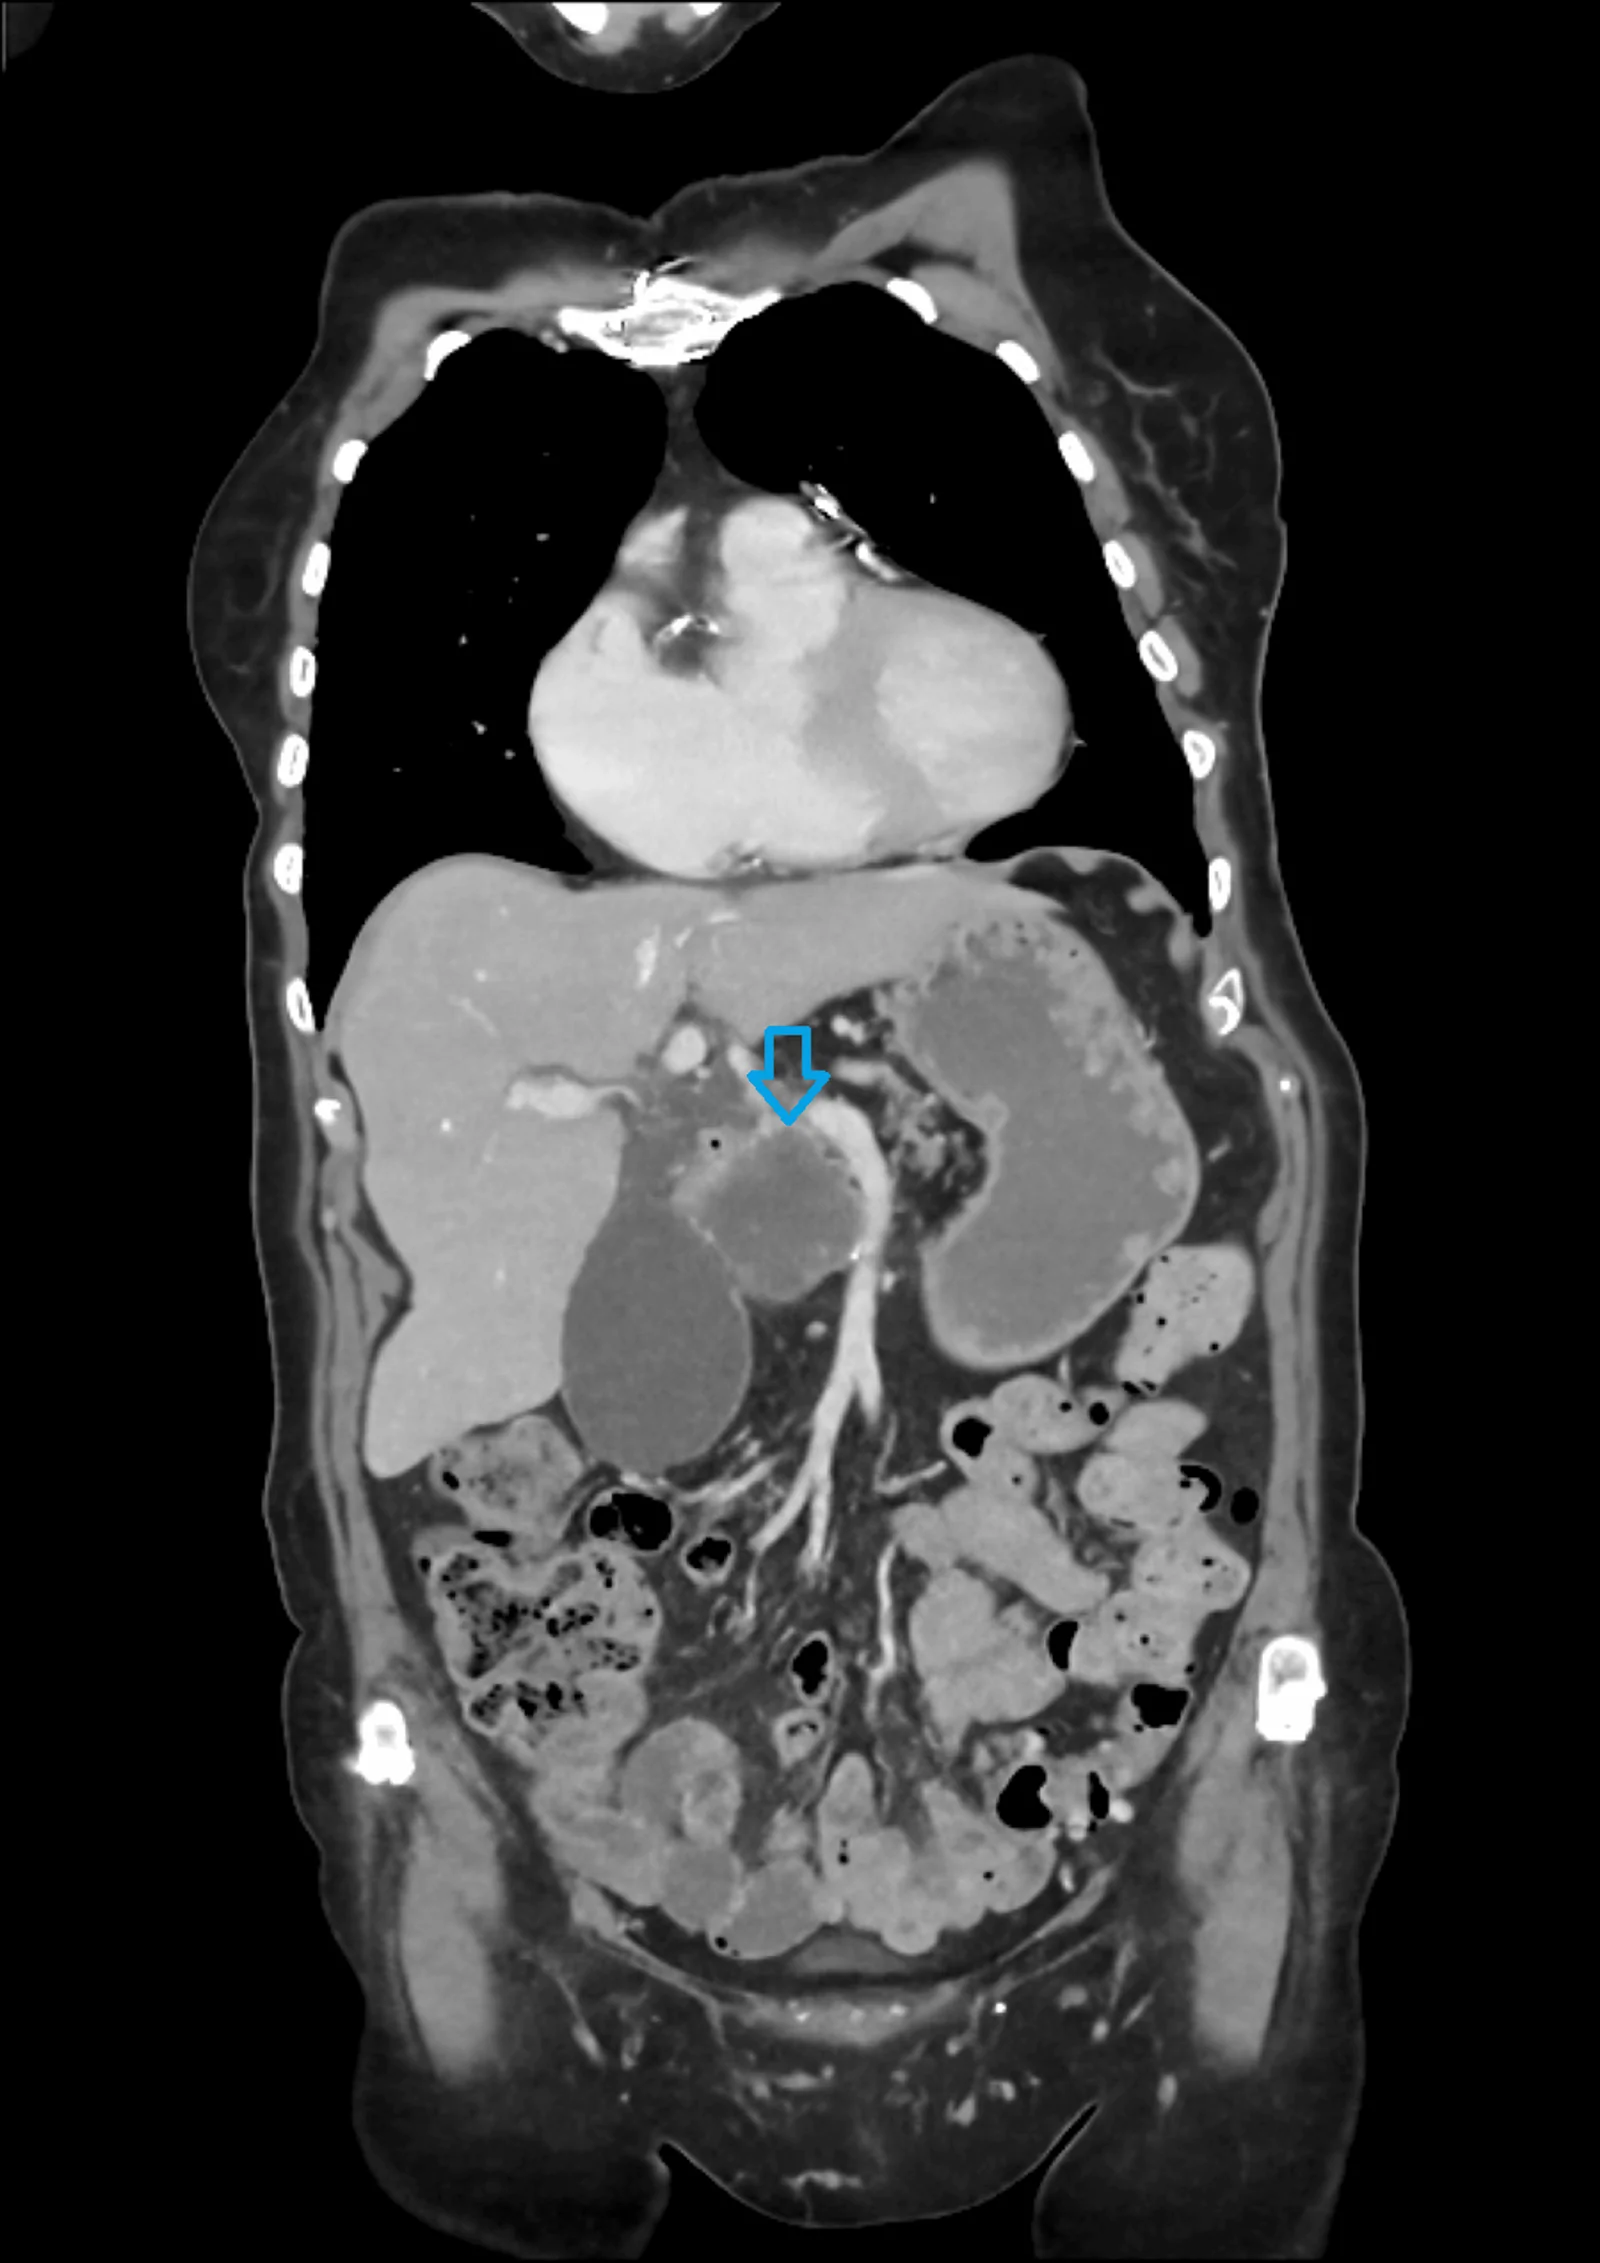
Coronal section of CT thorax, abdomen and pelvis with contrast, with the blue arrow pointing towards large pancreatic mass.
